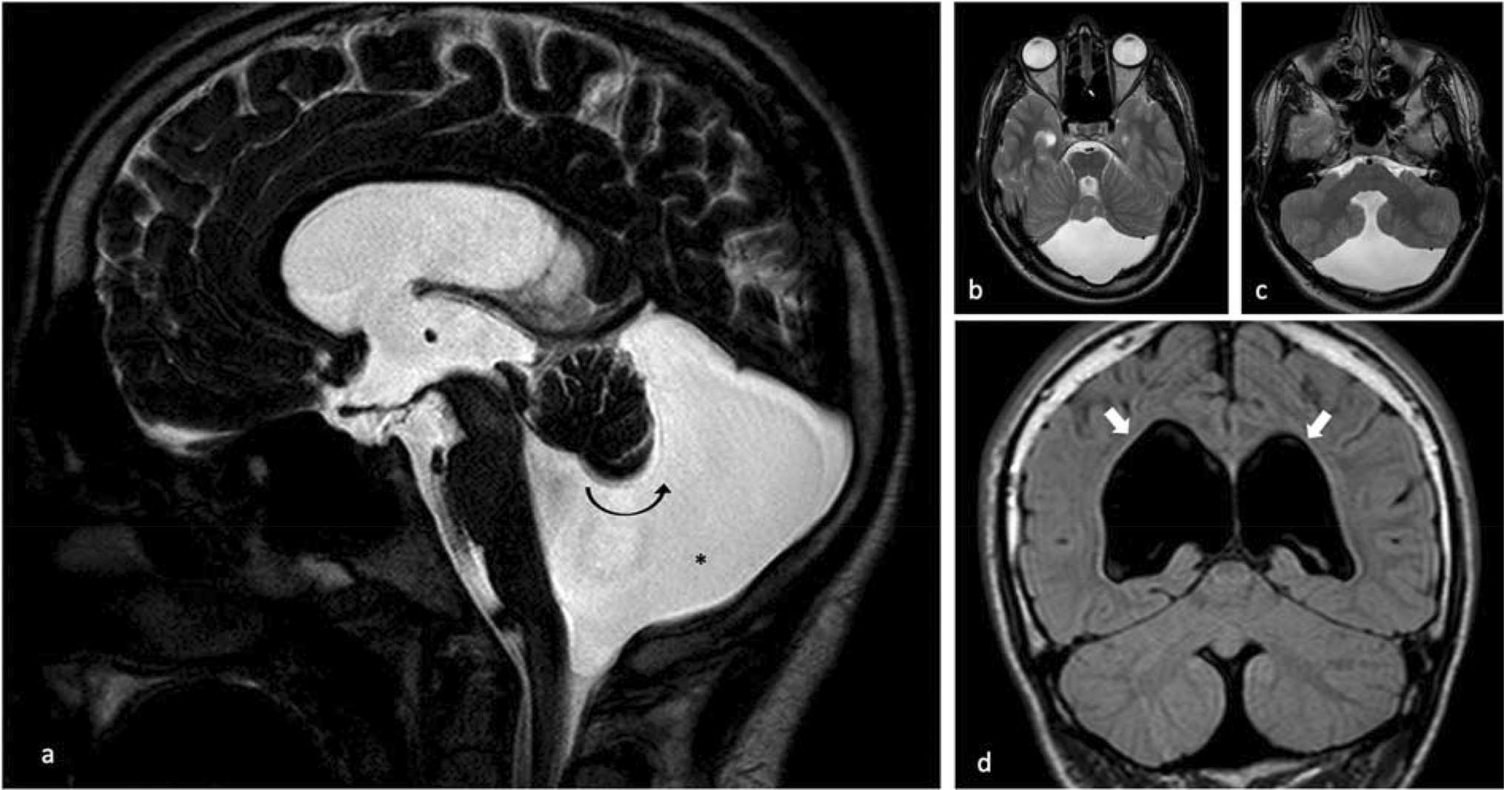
MRI of a 15-year-old boy with Dandy–Walker Variant. Sagittal 3D-DRIVE (a) Axial T2W (b, c) and coronal T1W (d) shows hypoplasia of the inferior vermis with anterosuperior rotation as seen on the sagittal scan (curved arrow), fourth ventricle dilatation and an enlarged posterior fossa (star). We can also see lateral ventricular dilatation (white arrows). There is no torcular-lambdoid inversion
